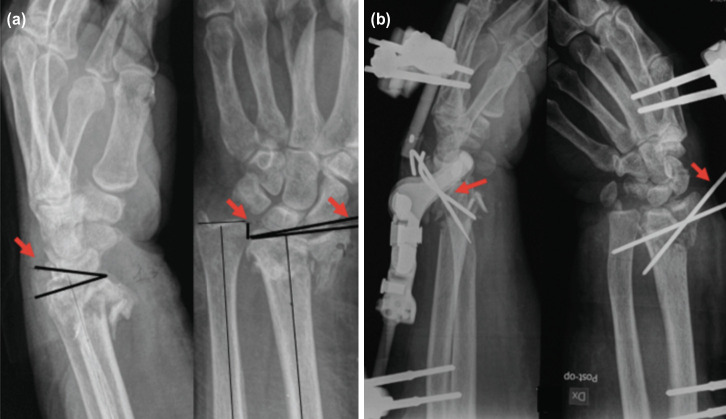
a) Pre-operative radiograph: the arrows showing angles for calculation of pre-operative radiological parameters. (b) Post-operative radiograph: the arrows showing use of K-wires to complement fracture reduction.
